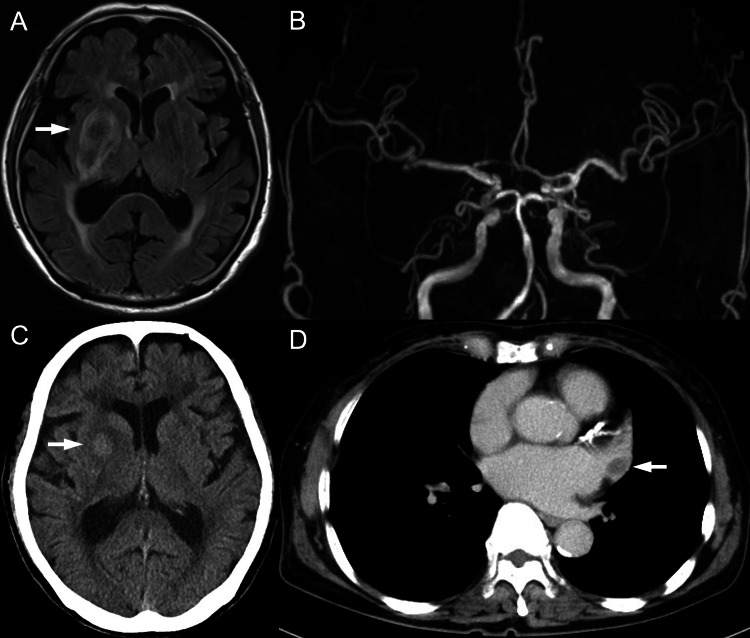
Post-procedural CT and MR images (A) MRI FLAIR sequence demonstrating an ischemic lesion with hemorrhagic transformation in the right basal ganglia (arrow). (B) MRA confirming persistent recanalization of the right ICA. (C) Head CT showing hemorrhagic changes in the right basal ganglia (arrow). (D) Contrast-enhanced chest CT revealing a filling defect in the LAA, consistent with thrombus (arrow). MRI: magnetic resonance imaging; FLAIR: fluid-attenuated inversion recovery; MRA: magnetic resonance angiography; ICA: internal carotid artery; LAA: left atrial appendage
